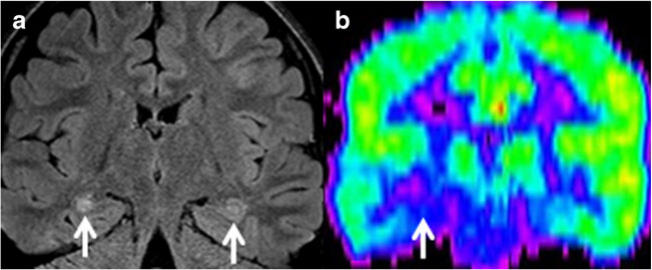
(a) Coronal FLAIR image of a 31-year-old man with partial complex seizures showing bilateral FLAIR hyperintensities in hippocampal body, right greater than left (*arrows*), suspected for bilateral mesial sclerosis. PET study (b), show unilateral hypometabolic focus (*arrow*), compatible with right-sided mesial temporal sclerosis
